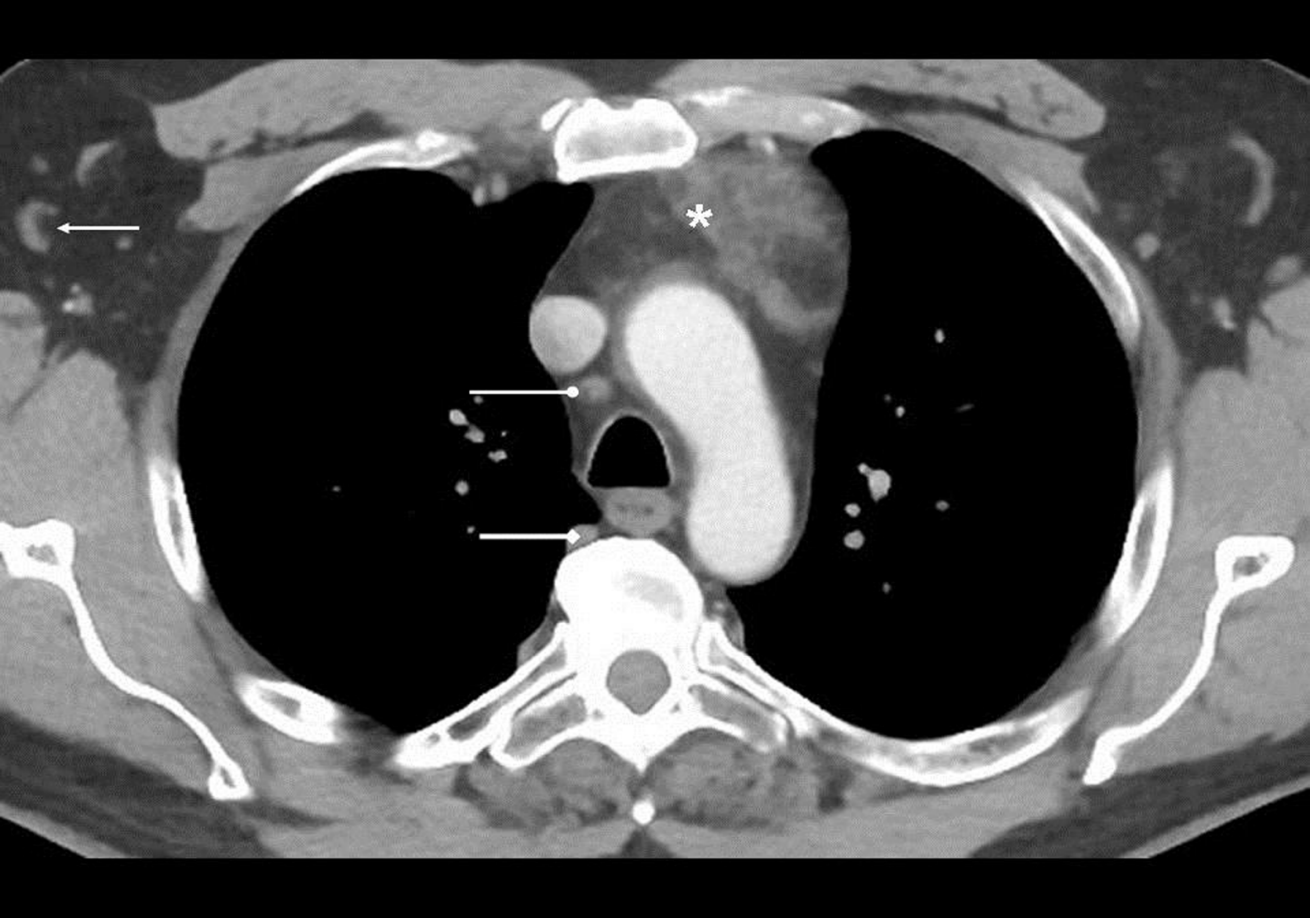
Contrast-enhanced CT of the upper chest in a 45-year-old man reveals a large heterogeneous anterior mediastinal mass (asterisk) with internal fatty density suggesting thymolipoma. A normal lymph node with a fatty hilum (arrow) is seen in the right axilla. Another normal sized lymph node (oval arrow) is seen in the right lower paratracheal region. The small circular soft tissue density seen in the right pre-vertebral region (diamond arrow) is azygous vein.
